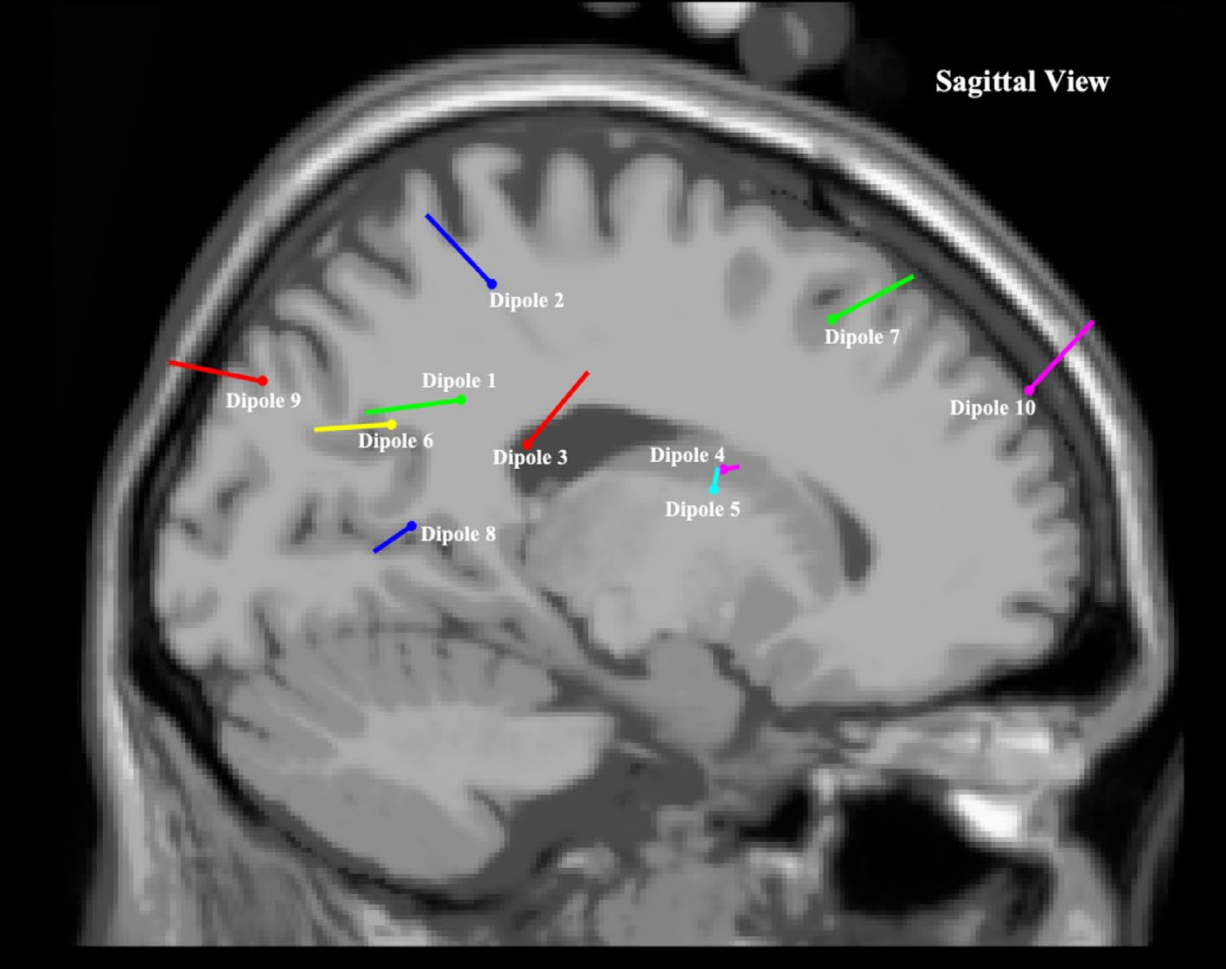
Sagittal view of most significant dipole locations superimposed on an averaged referenced MRI.
